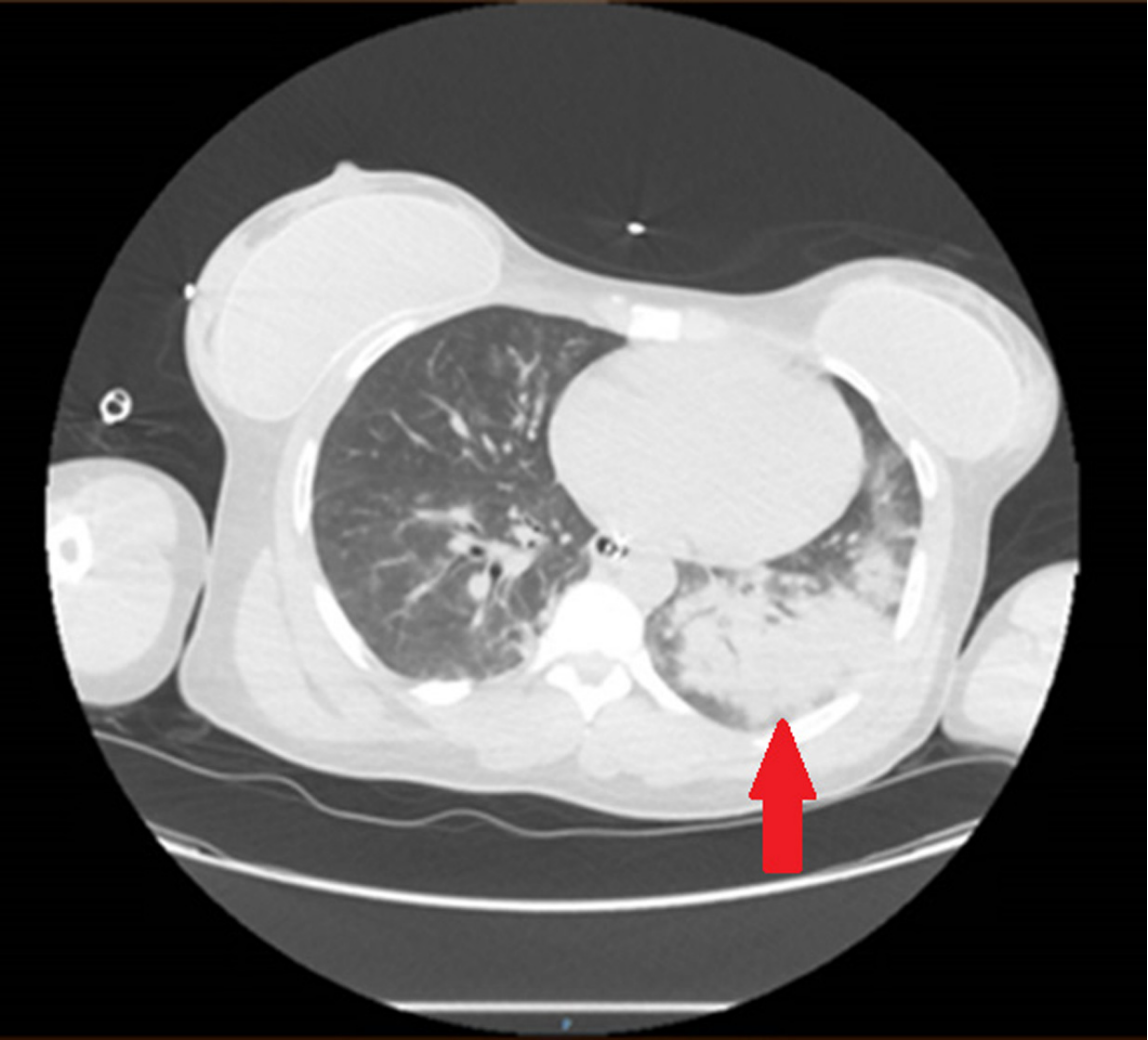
Computed tomography of the chest with contrast demonstrating bilateral pulmonary airspace disease, more pronounced in the left lung (arrow).
